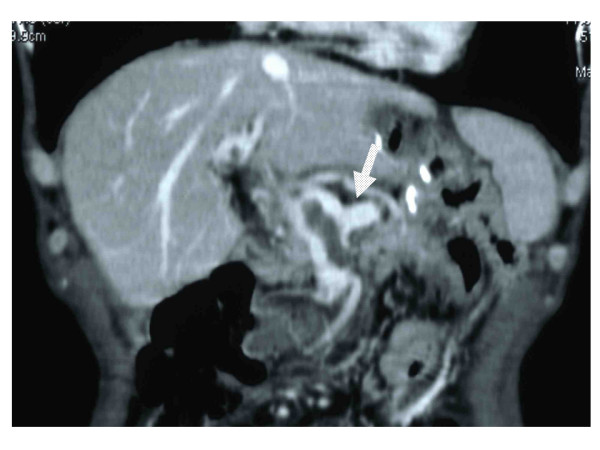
Contrast-enhanced abdominal CT. No thrombosis was observed in the splenic vein (arrow).
